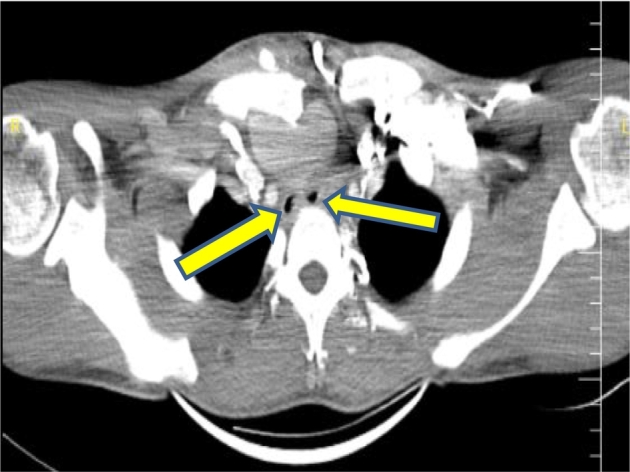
Computed tomography angiography with reconstruction revealed a pseudo-aneurysm of the brcahiocephalic artery and severe compresion in the main trachea.
